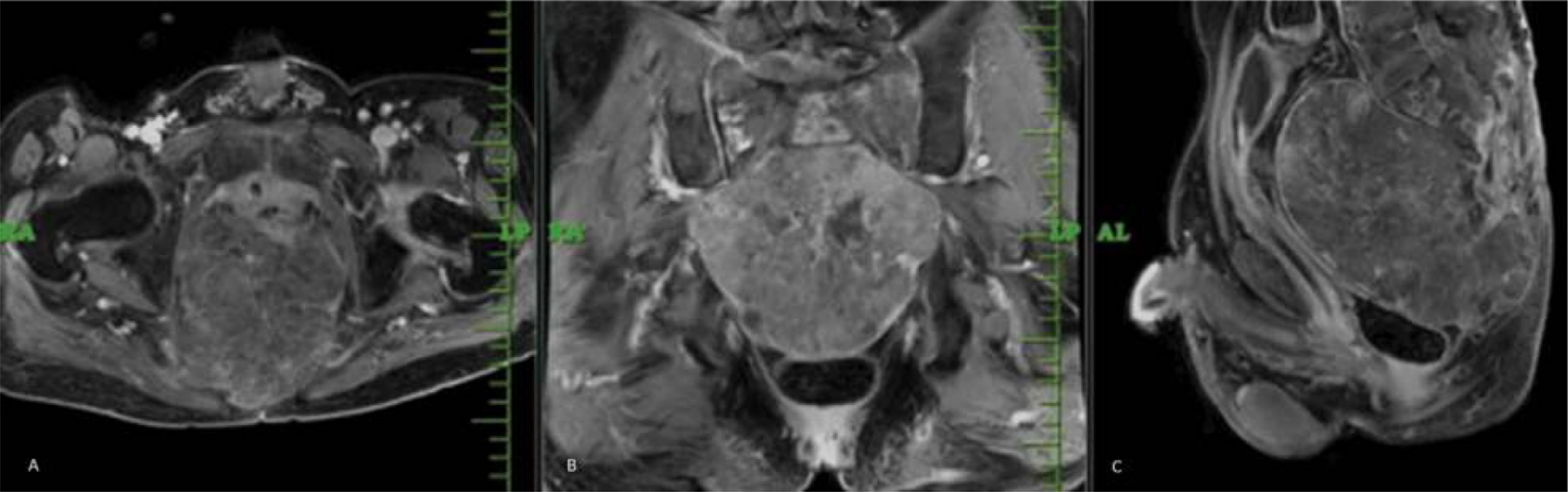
MRI post contrast axial(A), coronal (B), sagittal (C) sections showing large heterogenously enhancing mass lesion involving lower sacral vertebra and coccyx.
